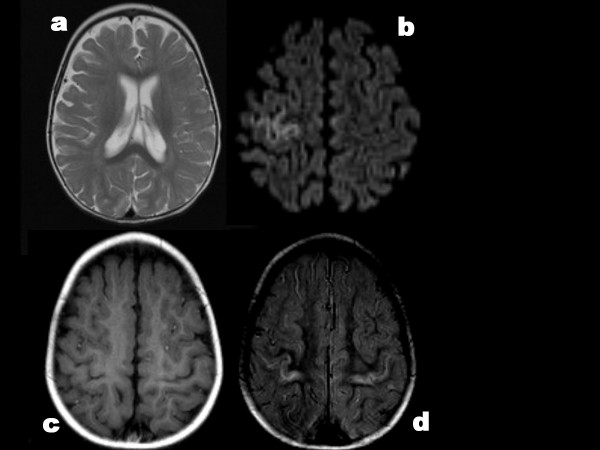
Patient 2, brain MRI. 2a: T2 weighted; mild tot moderate dilatation of ventricles and peripheral liquor spaces suggesting cerebral atrophy. 2b: TRACE sequence shows cytotoxic oedema in cortex of right central gyrus. 2c: 3 months later. Focal cortical atrophy of central gyrus bilaterally. 2d: 2 years later. Focal cortical atrophy of central gyrus bilaterally.
